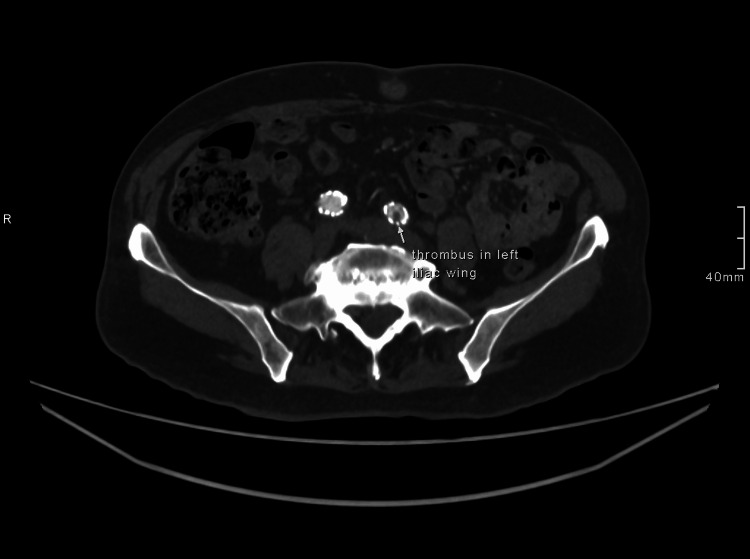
CT abdominal aorta with contrast showing thrombus in the left iliac wing of the graft
